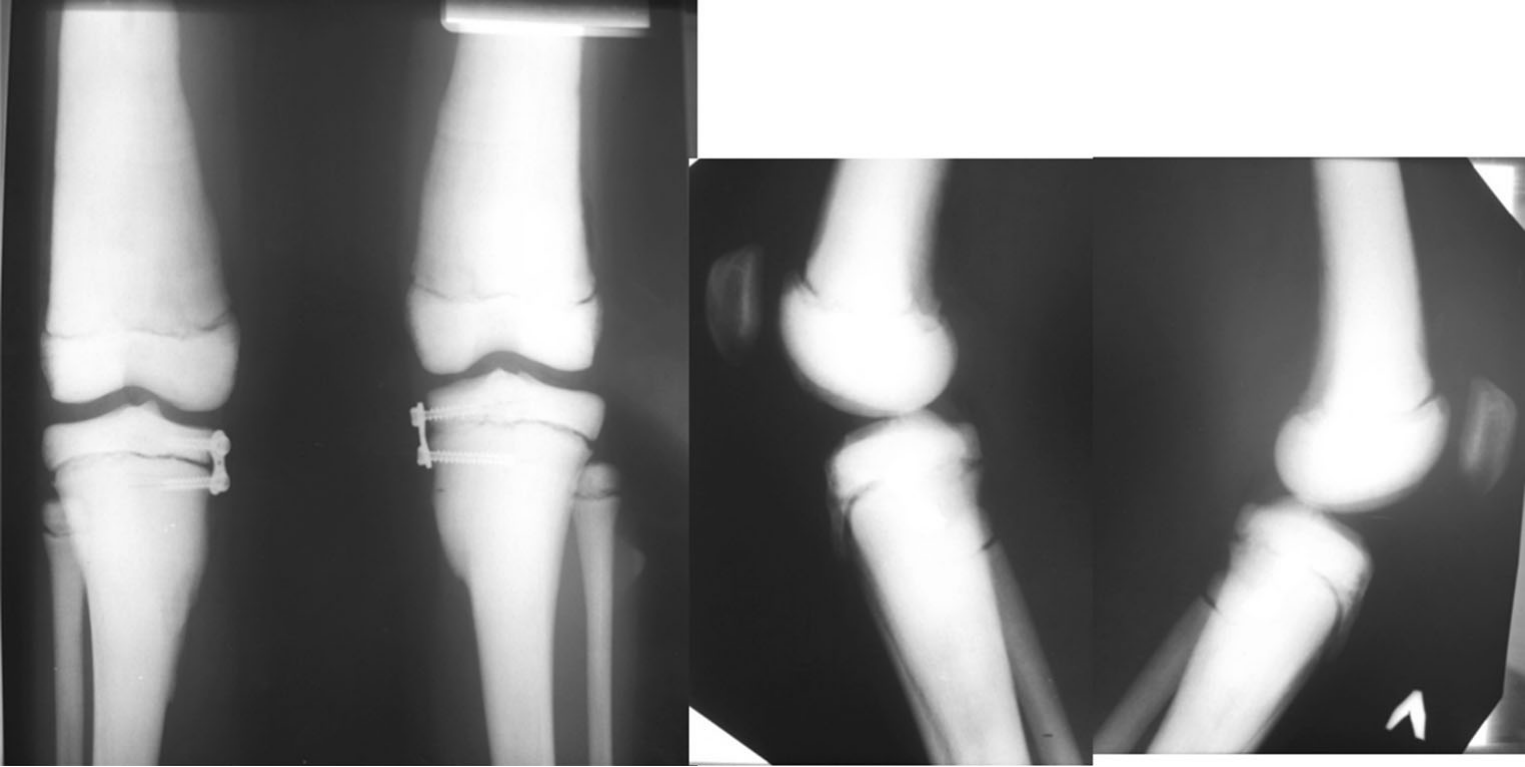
AP and lateral knee radiographs of lower limbs after surgery
